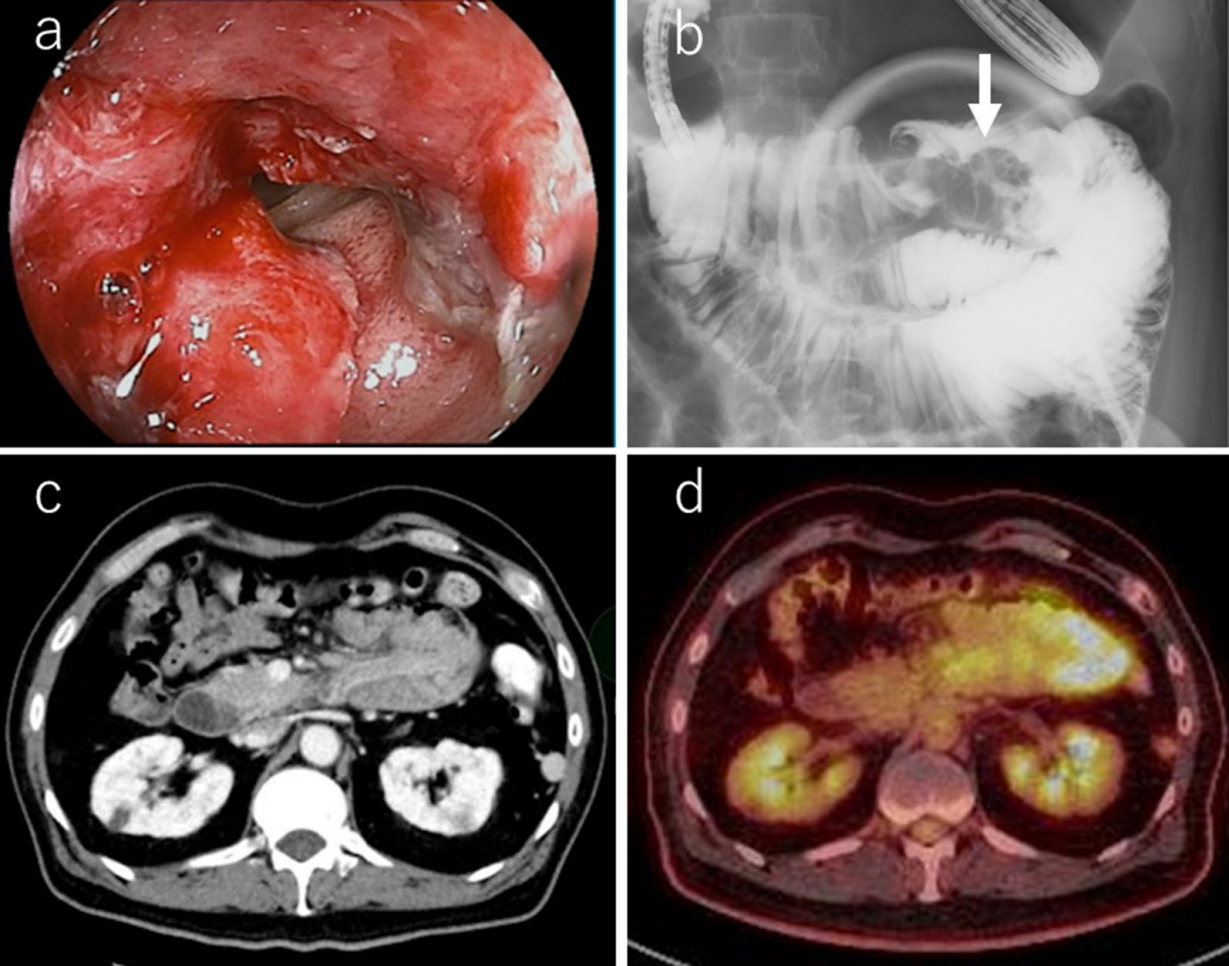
Preoperative imaging findings. a Endoscopic findings: enteroscopy revealed an easily hemorrhagic tumor in the jejunum. b Findings of the small bowel series using meglumine sodium amidotrizoate: a tumor (white arrow) was found in the jejunum at the angle of Treitz. c Contrast-enhanced computed tomography findings: The tumor with intussusception appeared as a reniform shape (pseudokidney sign) longitudinally and demonstrated multilayered concentric rings of mass (target sign) transversely. A tumor with intussusception and regional lymph node swelling was located at the angle of Treitz. d Findings of the fluorodeoxyglucose positron emission tomography: this tumor had marked hypermetabolism, showing a standardized uptake value (SUV) max of 8.3, and the swollen regional lymph node showed mild hypermetabolism with a SUV max of 3.7
